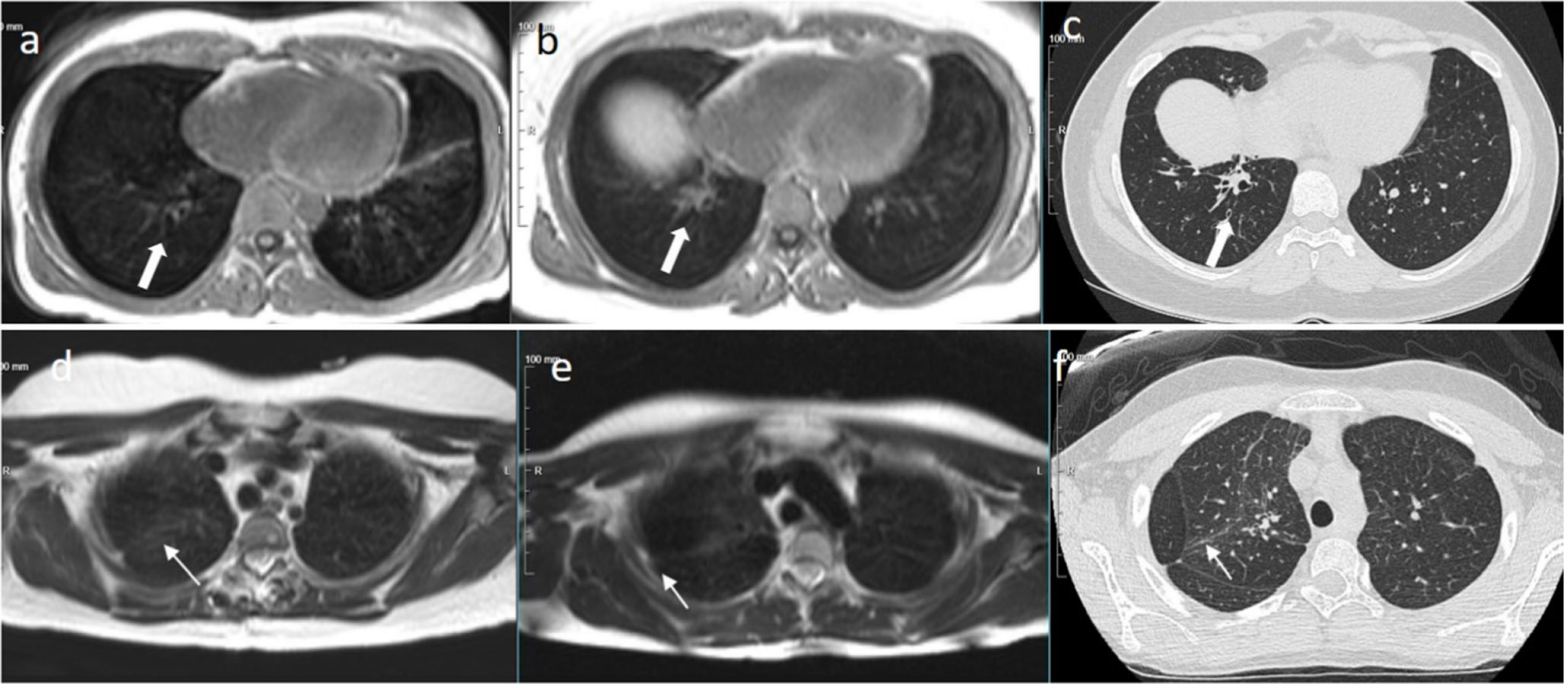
Pulmonary imaging of two study participants. Upper row: patient 6 with stable bronchiectasis (thick arrows) of the lower lobes; a the recent MRI (axial 3DFFE sequence) and 6 years earlier obtained, b baseline MRI (axial 3DFFE sequence), and c HRCT. Lower row: patient 2 with apical pleural thickening, parenchymal stranding and scarring (thin arrows), but no bronchiectasis. No progression of findings from baseline in HRCT or MRI imaging; d the recent MRI (axial T2 TSE sequence) and 6 years earlier obtained, e baseline MRI, and f HRCT
